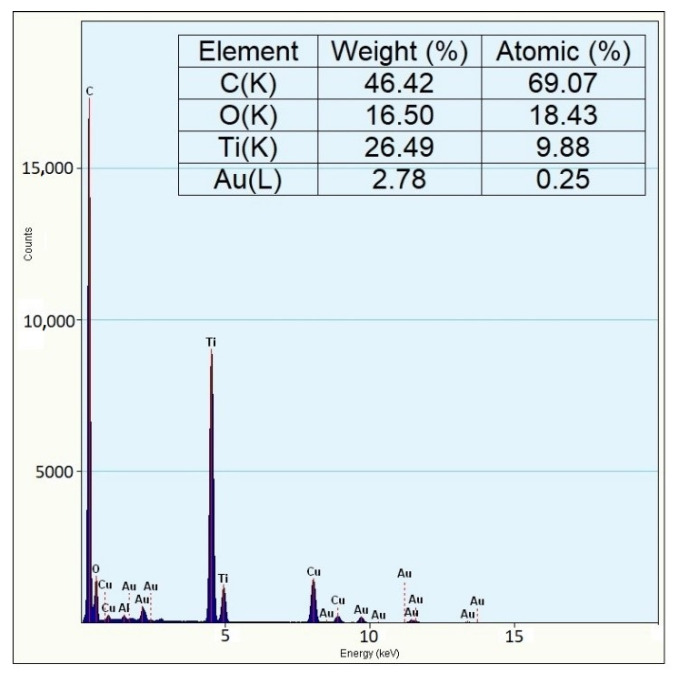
Energy dispersive X-ray (EDX) image for the as-synthesized TiO_2_/Au NSs.
